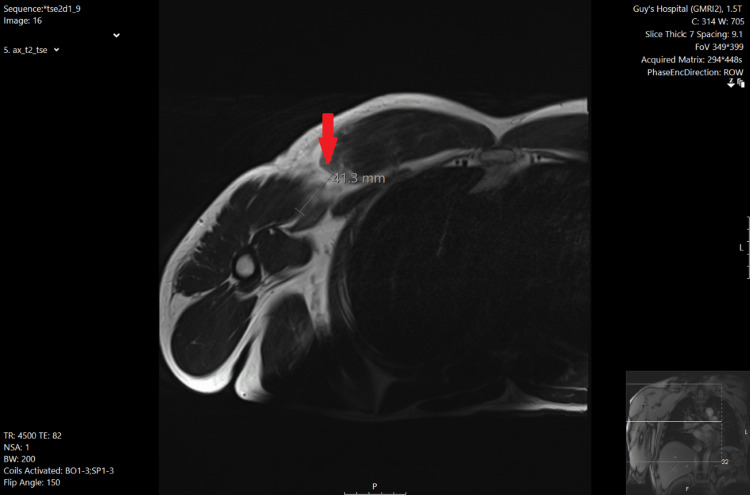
Right pectoralis major MRI scan Arrow demonstrating the PM rupture Distance between ruptured free edges (retraction) is measured 41.3mm
